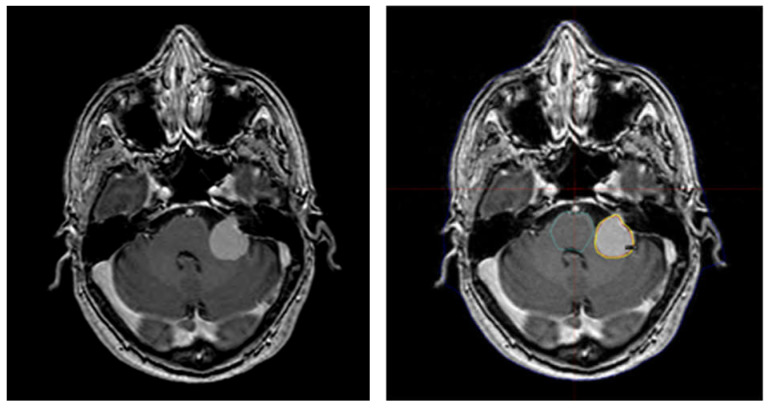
Left: MRI showing the enhancement post-gadolinium administration. Right: MRI of the brain to contour the tumor on 1.5T clinical scanners (Philips Achiva, Best, The Netherlands) used in the planning stage with gamma plan station. Images were transferred to the gamma plan working station. The tumor showed a volume of 5514 cm^3^. Treatment was delivered by the Leksell Gamma Knife Icon^TM^ (Elekta, Stockholm, Sweden) as a single dose with 13 Gy marginal dose, 50% isodose.
